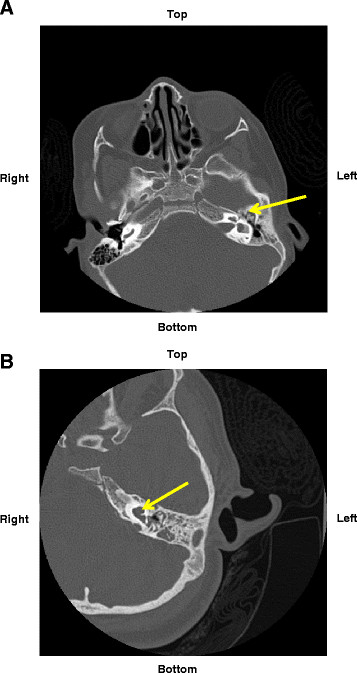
CT scans of inner ear cavities and adjacent sinuses performed at Texas Children’s Hospital. A, Case Presentation 1 showing diminished left middle ear cavity and mastoid sinus (yellow arrow); B. Case Presentation 2 showing a dysplastic cochlea with an abnormally large basal turn and only partially formed middle and to a lesser extent apical turns (yellow arrow). The vestibule was also dysplastic (not shown).
